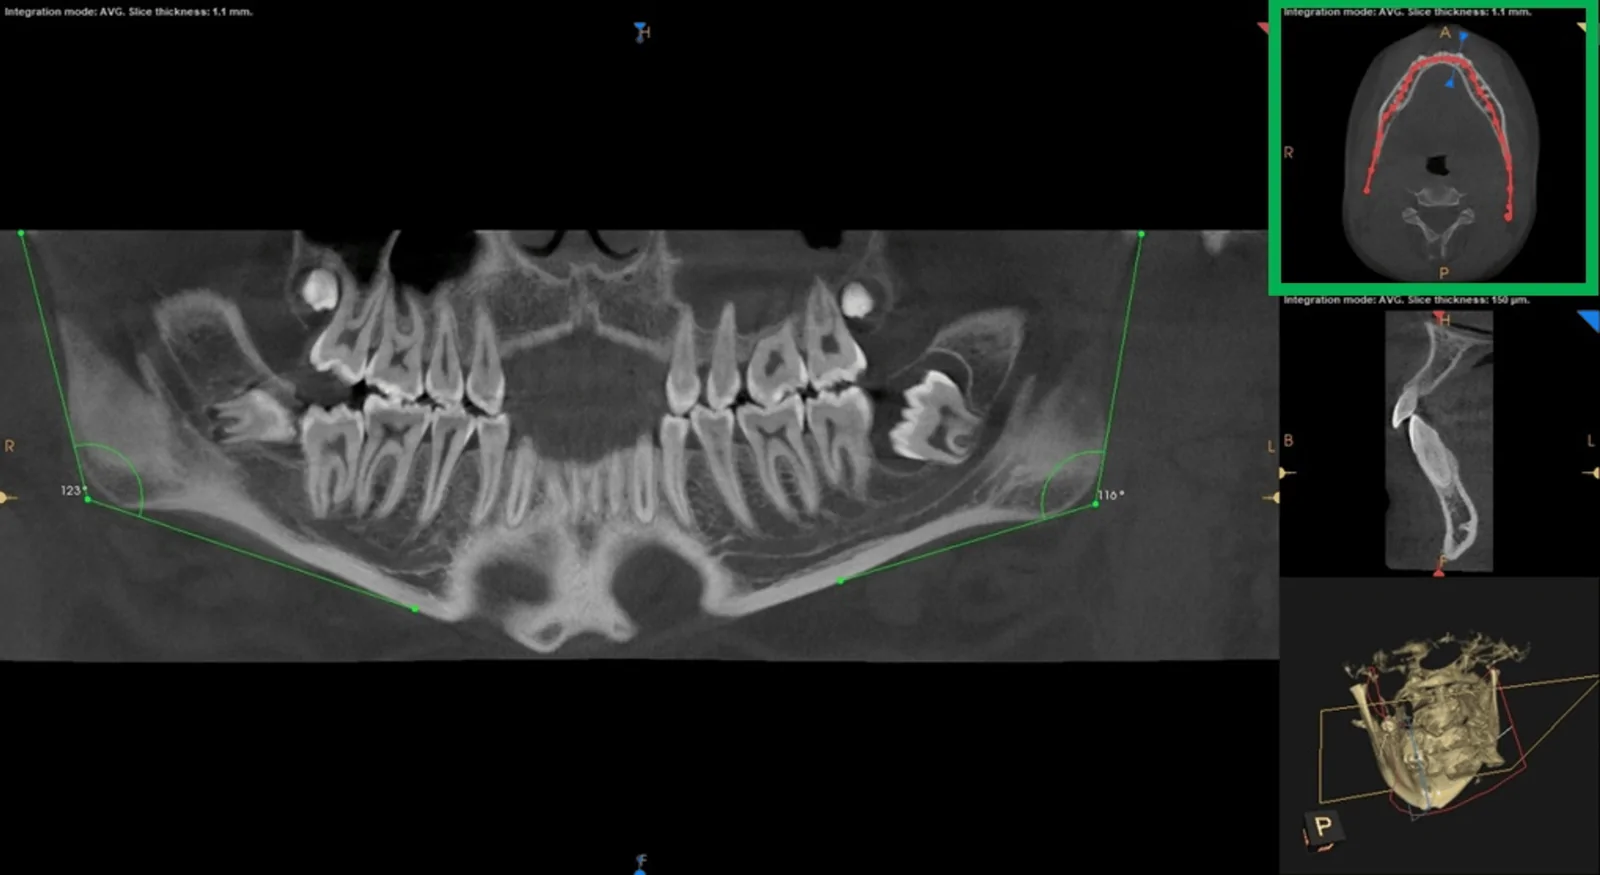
CBCT image of the mandible showing the right and left gonial angles (Ar-Go-Me; green), measured bilaterally between the Ar-Go line along the posterior border of the ramus and the Go-Me line along the inferior border of the mandible. Ar: Articulare; CBCT: Cone-beam computed tomography; Go: Gonion; Me: Menton.
